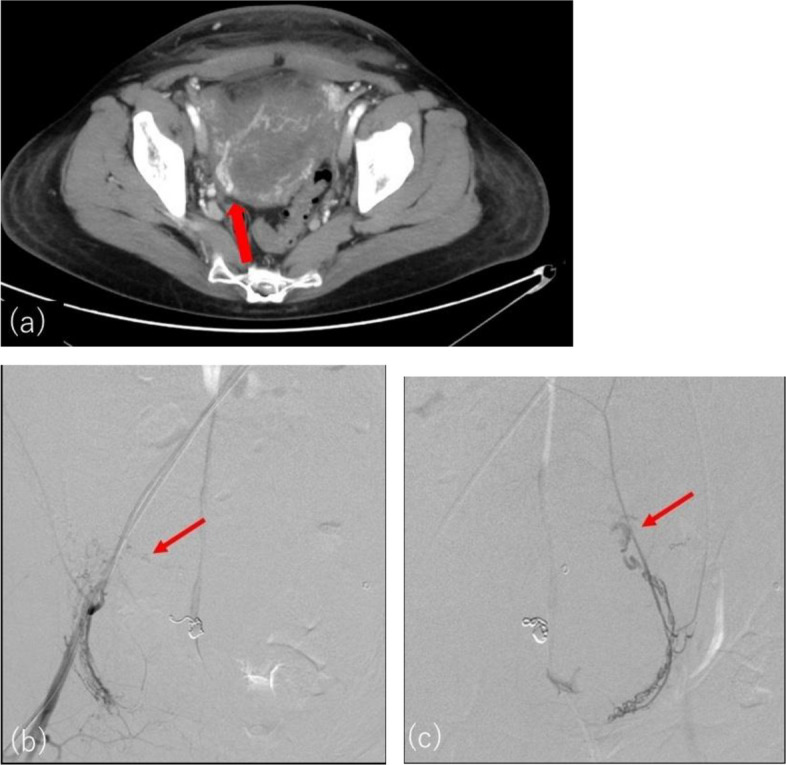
Severe postpartum hemorrhage due to retained placenta in a 31-year-old woman. Contrast-enhanced computed tomography demonstrated periuterine hematoma near the round ligament with active extravasation (arrow) (a). Bilateral uterine arteries were embolized with gelatin sponge and coils; however, hemodynamic instability persisted. b, c Angiography demonstrated active contrast extravasation from the bilateral round ligament arteries arising from the inferior epigastric arteries (arrows). Additional transcatheter arterial embolization was performed using gelatin sponge and coils
